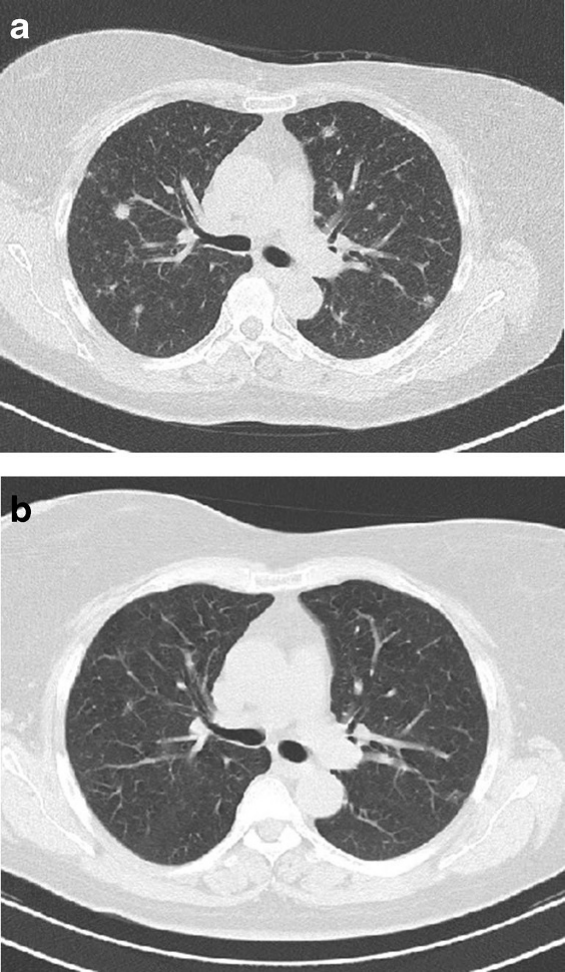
A 61-year-old woman, smoker, with asymptomatic PLCH and bone involvement. Diagnosis obtained by scapular biopsy. a A 3-mm collimated CT demonstrates nodular infiltrates with irregular borders. Some minimal faint cysts are also appreciable (*arrow*). b A follow-up CT scan 6 months post-diagnosis shows almost complete regression of pulmonary nodules. Improvement occurs with smoking reduction, but not cessation
